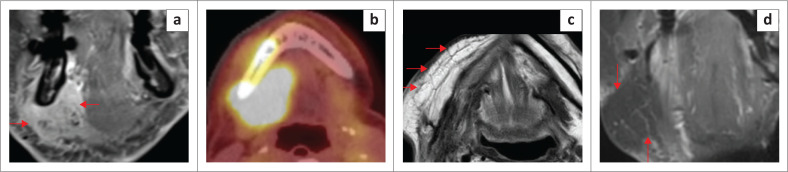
An elderly patient with a malignant tumour in the right FOM and submandibular region as seen on the T1 coronal post contrast (a). The lesion is markedly avid on ^18^F-fluorodeoxyglucose positron emission tomography/computed tomography (^18^F-FDG PET/CT) (b). The T2 axial (c) shows the post-operative appearance after right mandibular resection and graft repair (bright signal of graft fat; red arrows). The contrast-enhanced fat-suppressed T1 weighted (T1W) coronal image (d) shows complete suppression of fat signal (red arrows) and mild oedema at the surgical site.
